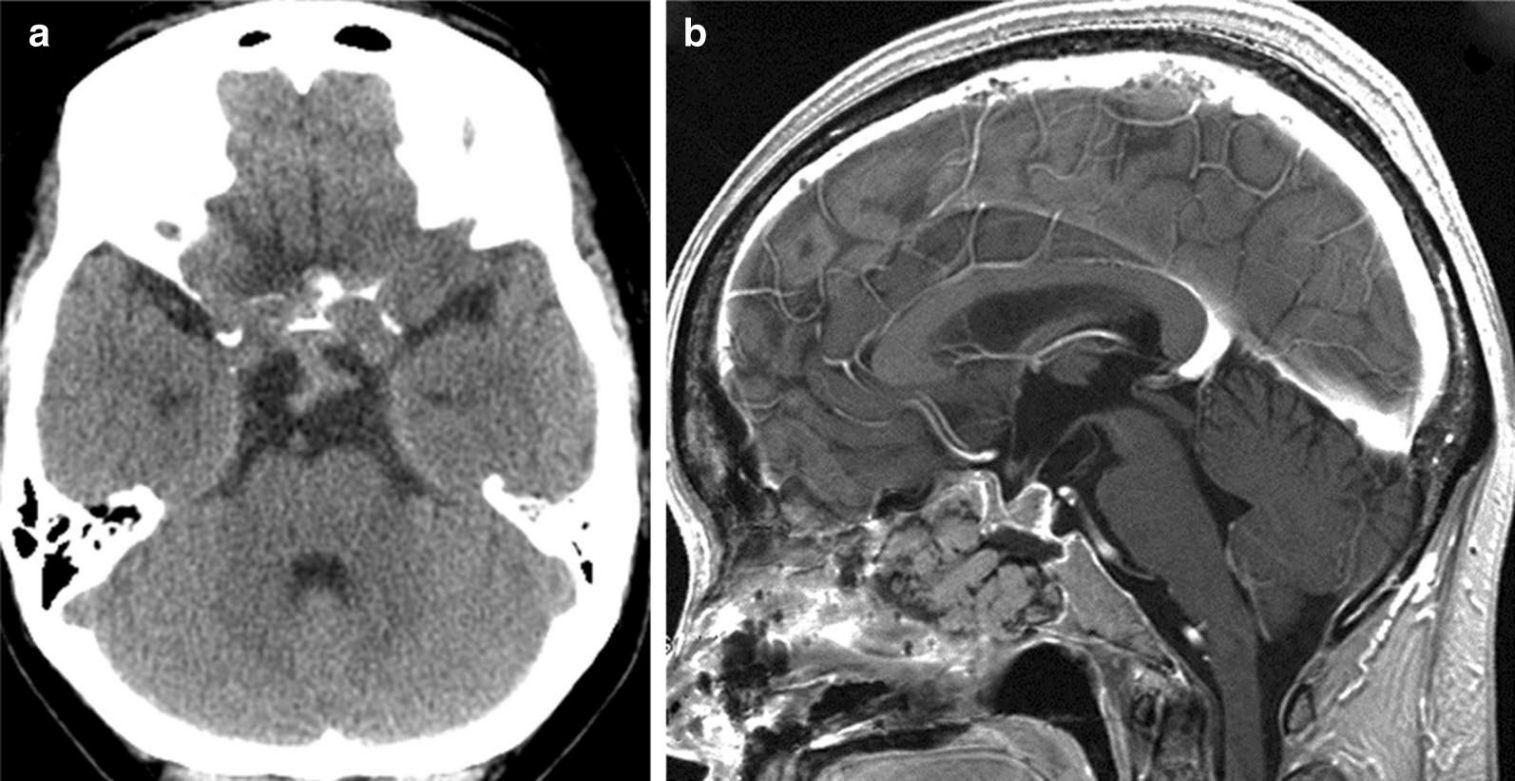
a Axial computed tomography image obtained immediately after the first transsphenoidal surgery showing no hemorrhagic complication. b: Sagittal contrast-enhanced T1-weighted magnetic resonance image obtained 3 days after the second transsphenoidal surgery showing subtotal removal of the tumor
